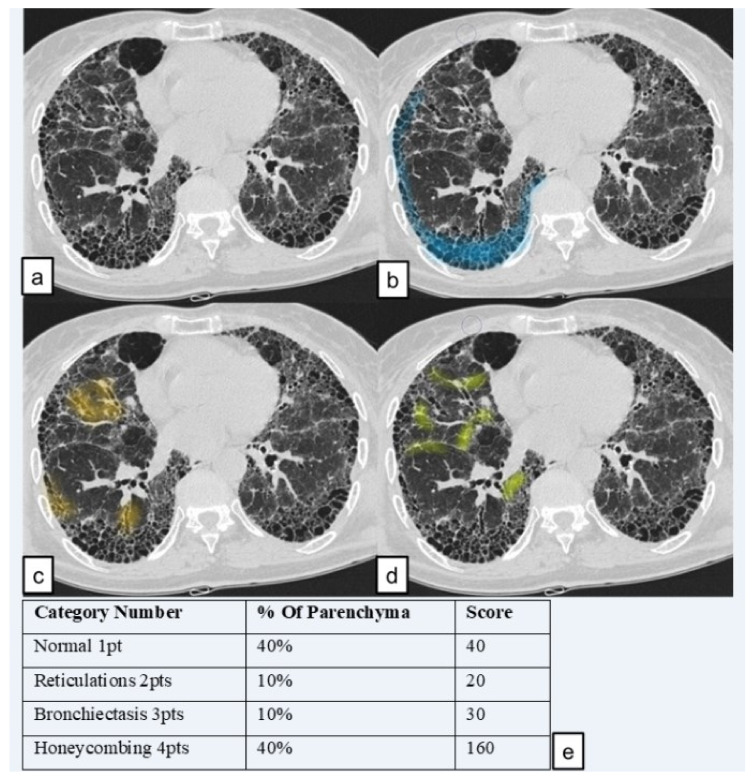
An example of HRCT score of “lower zone” of the right lung. (a) Slice of “lower zone” (b) in blue, area of honeycombing (c) in orange, area with traction bronchiectasis; (d) area of reticulation. (e) Scores of the slice (obtained by multiplying the category number with the affected percentage area).
